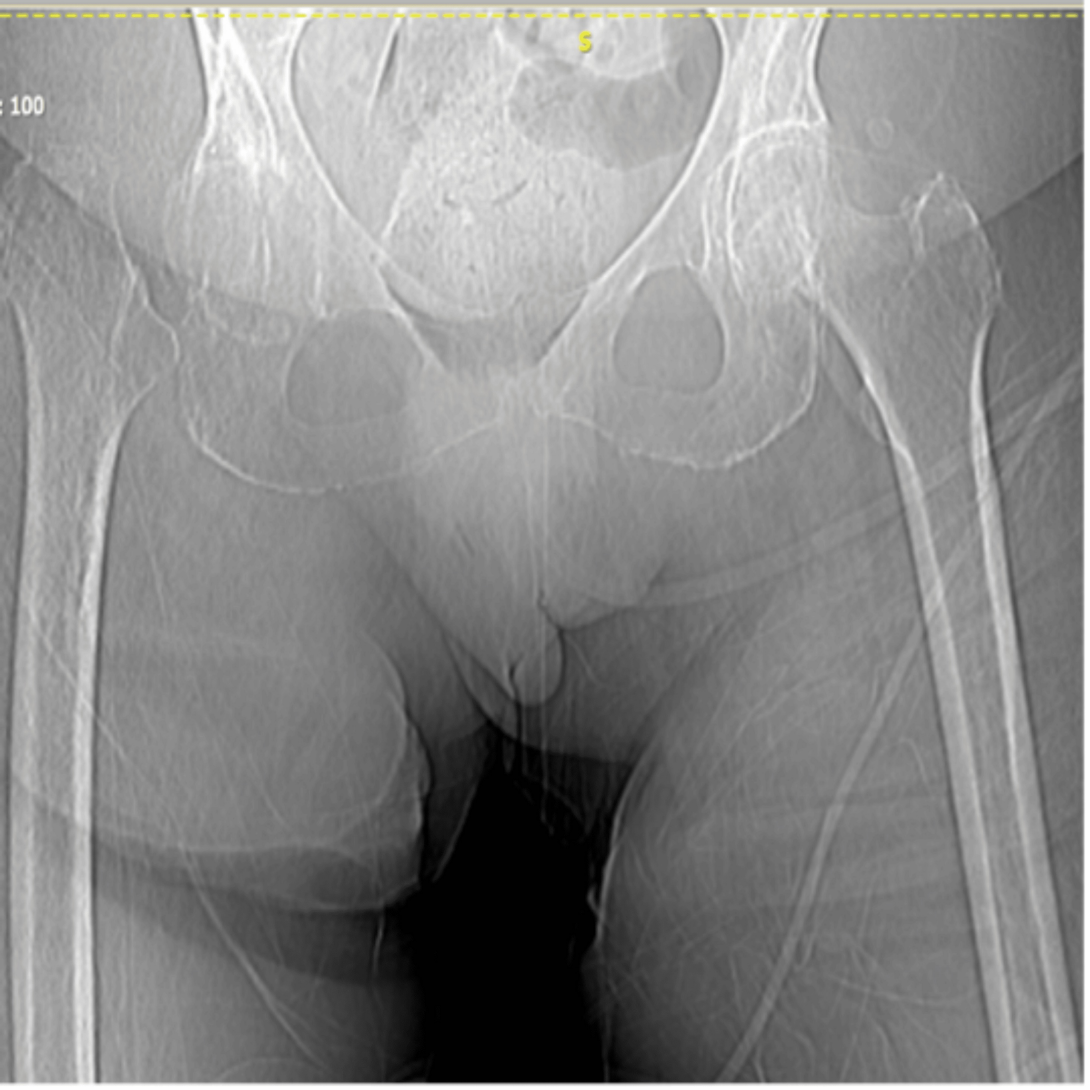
Plain radiograph during mid-term follow-up
